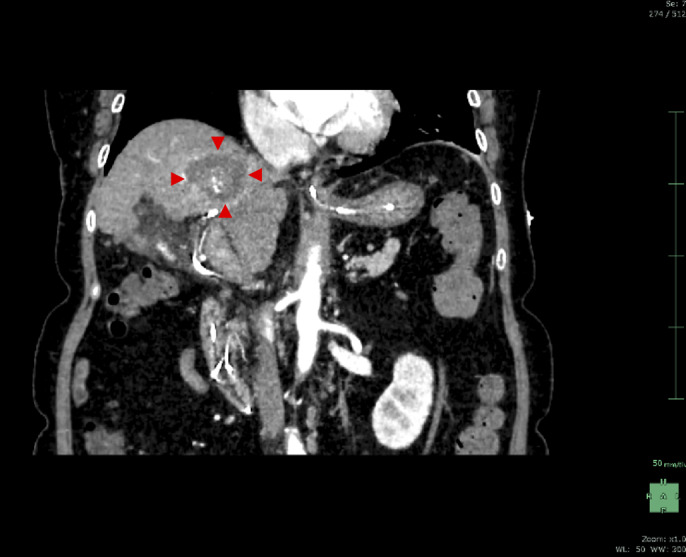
Follow-up computed tomography scan showing complete ablation of the hepatocellular carcinoma (red arrowheads).
